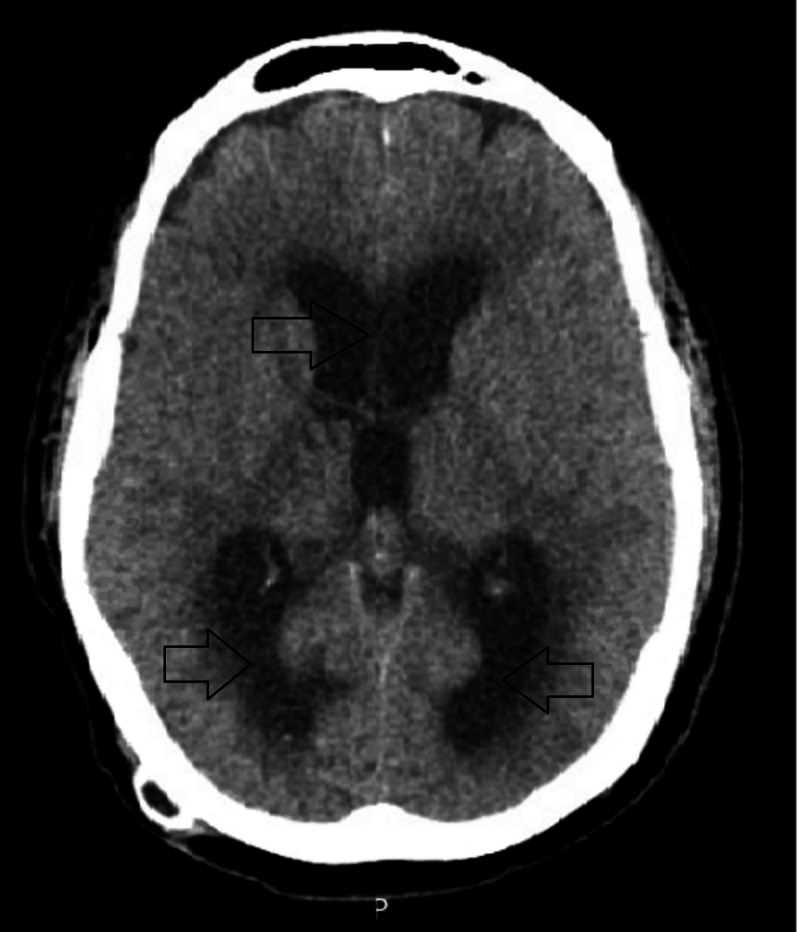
Computed tomography showing hydrocephalus that involves the lateral and third ventricles
